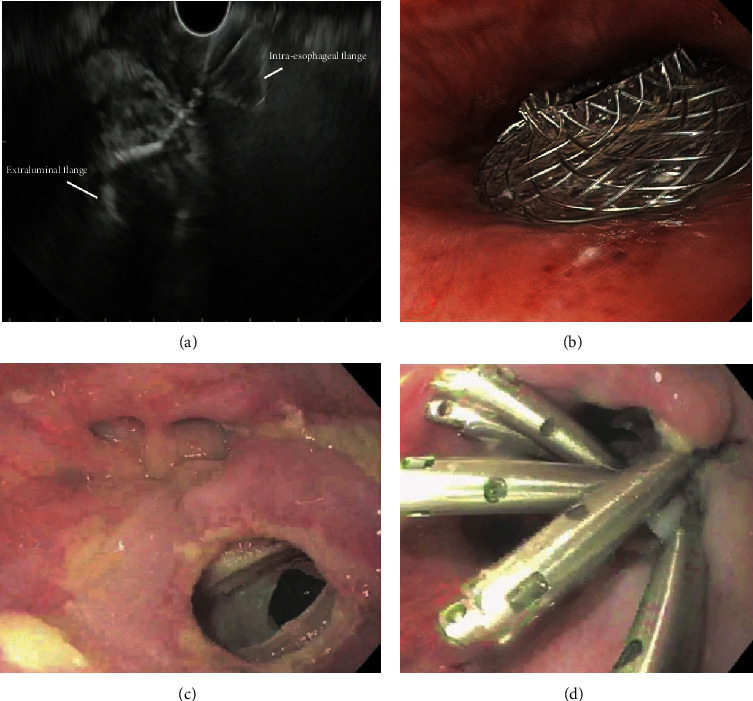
Patient with a mediastinal collection adjacent to the esophagus. (a) Endosonographic image showing deployment of a 10 mm diameter lumen apposing metal stent (LAMS). (b) Endoscopic image of the proximal flange placed in the esophagus. (c) Endoscopic image of the esophageal defect after LAMS removal. (d) Endoscopic image of the esophageal defect closed with endoclips.
